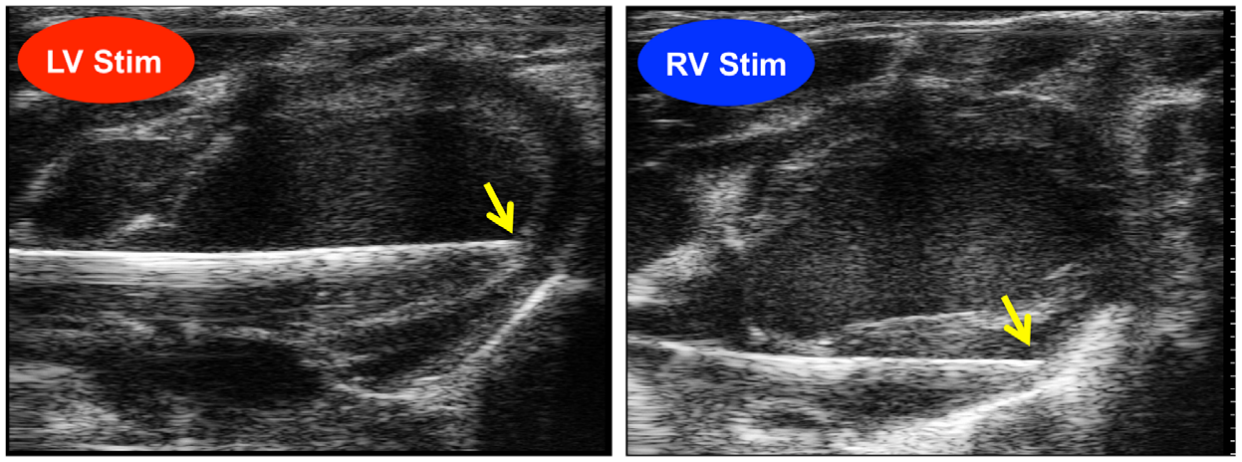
Representative echocardiograms of the electrical stimulation catheter inside the left (LV) or right ventricle (RV). Yellow arrowheads indicate the tip of the catheter from which electrical pulses were administered. The tip of the catheter was positioned at the interventricular septum in LV and the free wall in RV.
